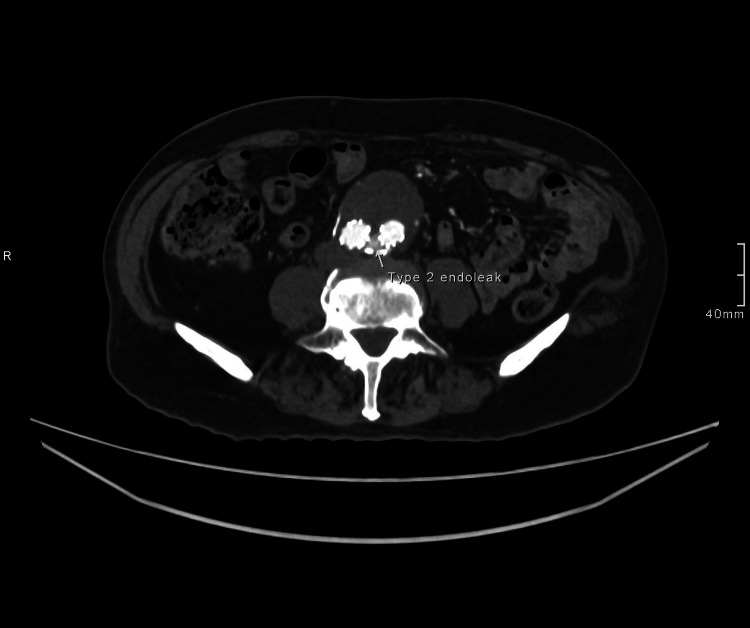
CT abdominal aorta with contrast showing type 2 endoleak
